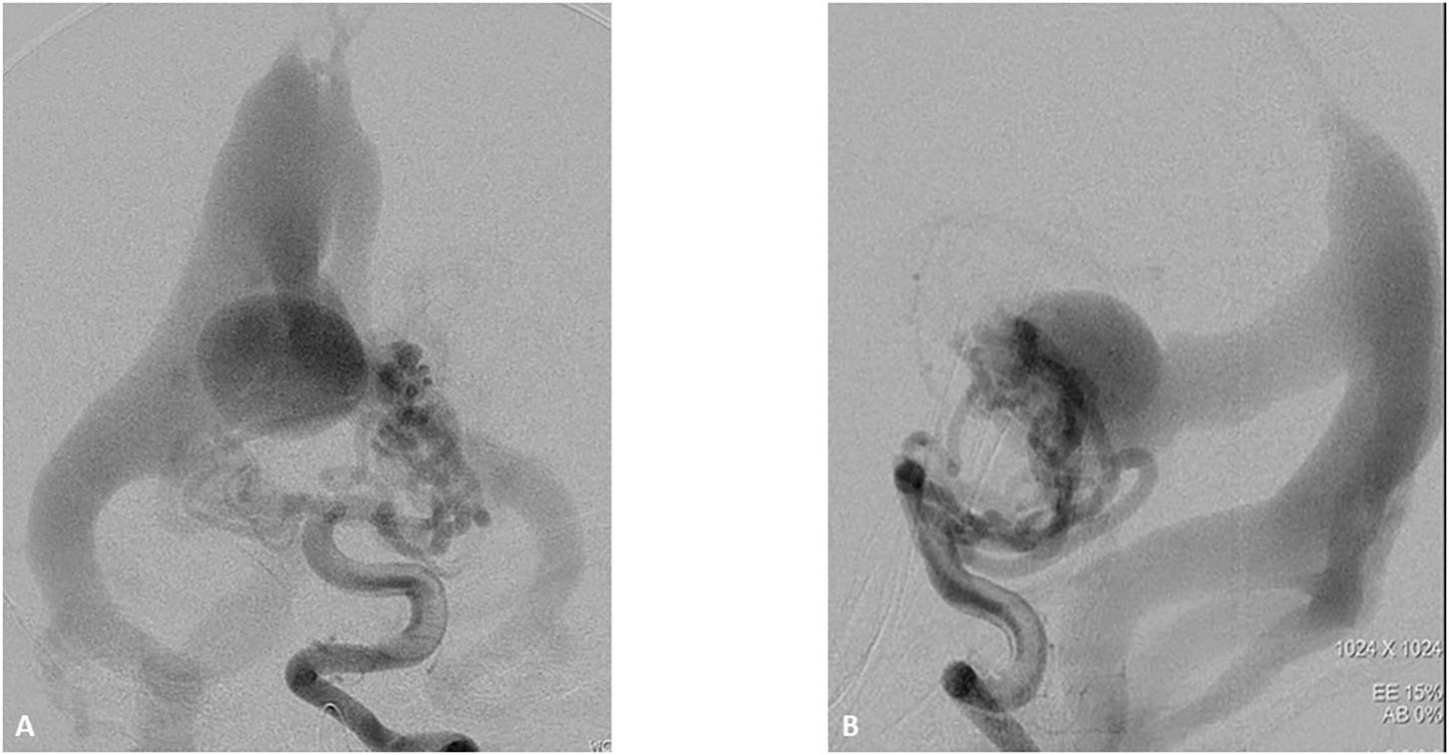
One-month boy presented with severe high failure requiring pressors and ICU management. Initial ultrasound through the frontal fontanelle showed concerns for a vein of Galen malformation. A vertebral DSA AP (A) and lateral (B) views reveal a high flow vein of Galen choroidal malformation with enlarged choroidal feeders of the posterior cerebral artery, left greater than right. These fistulae drain into a dilated vein of Galen and median vein of the prosencephalon. There is filling of the bilateral transverse sigmoid sinuses, right greater than left as well as some opacification of the occipital.
